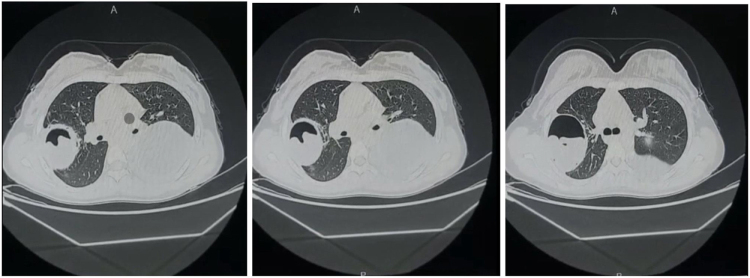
CT of the chest shows bilateral lung cysts, the one at the left side is intact, round, homogeneous, and water-dense with an enhancing rim. The cyst at the right upper lobe is a ruptured cyst; the CT shows air-fluid level with crumpled endo-cyst membrane appearing as floating membrane.
